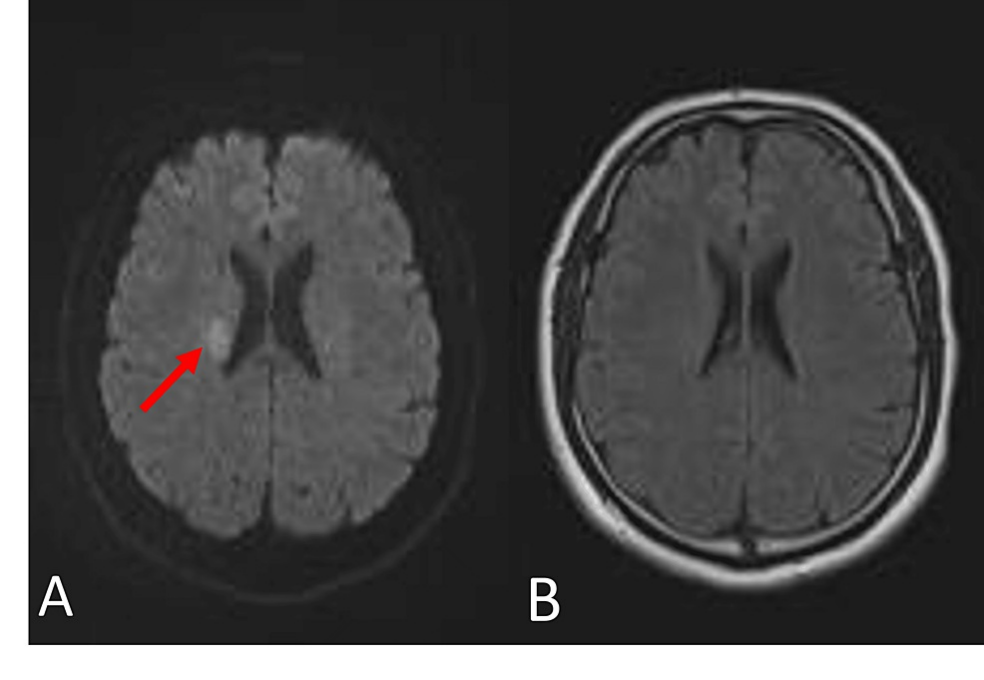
MRI of the brain showing “mismatch” between (A) axial DWI demonstrating a small focus of high signal intensity in the right internal capsule and putamen (red arrow) and (B) FLAIR image with no visible signal change.
